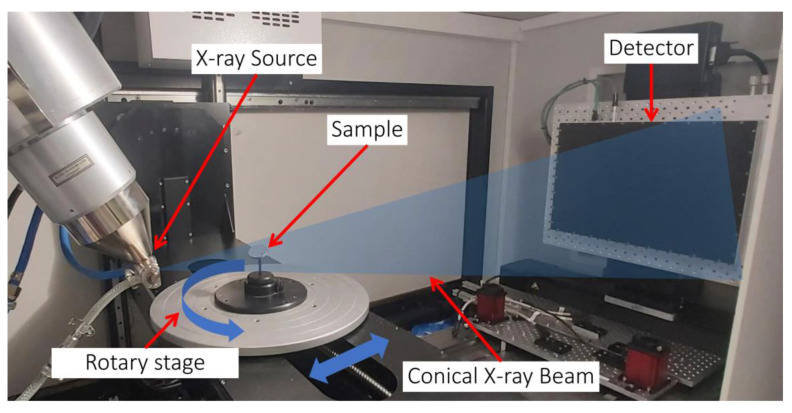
Industrial 3D X-ray computed tomography setup.
